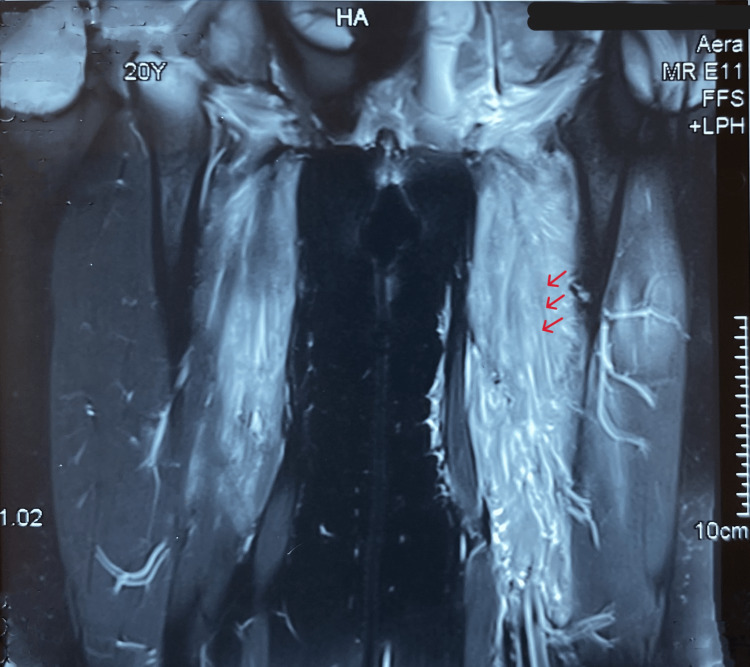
MRI of the thighs shows an inflammatory myositis of the thighs, with edematous infiltration of the muscles of the two thighs involving the short and long adductor muscles and the left quadriceps extending to the intermuscular fascias in hyposignal T1 and hypersignal T2. (red arrows)
